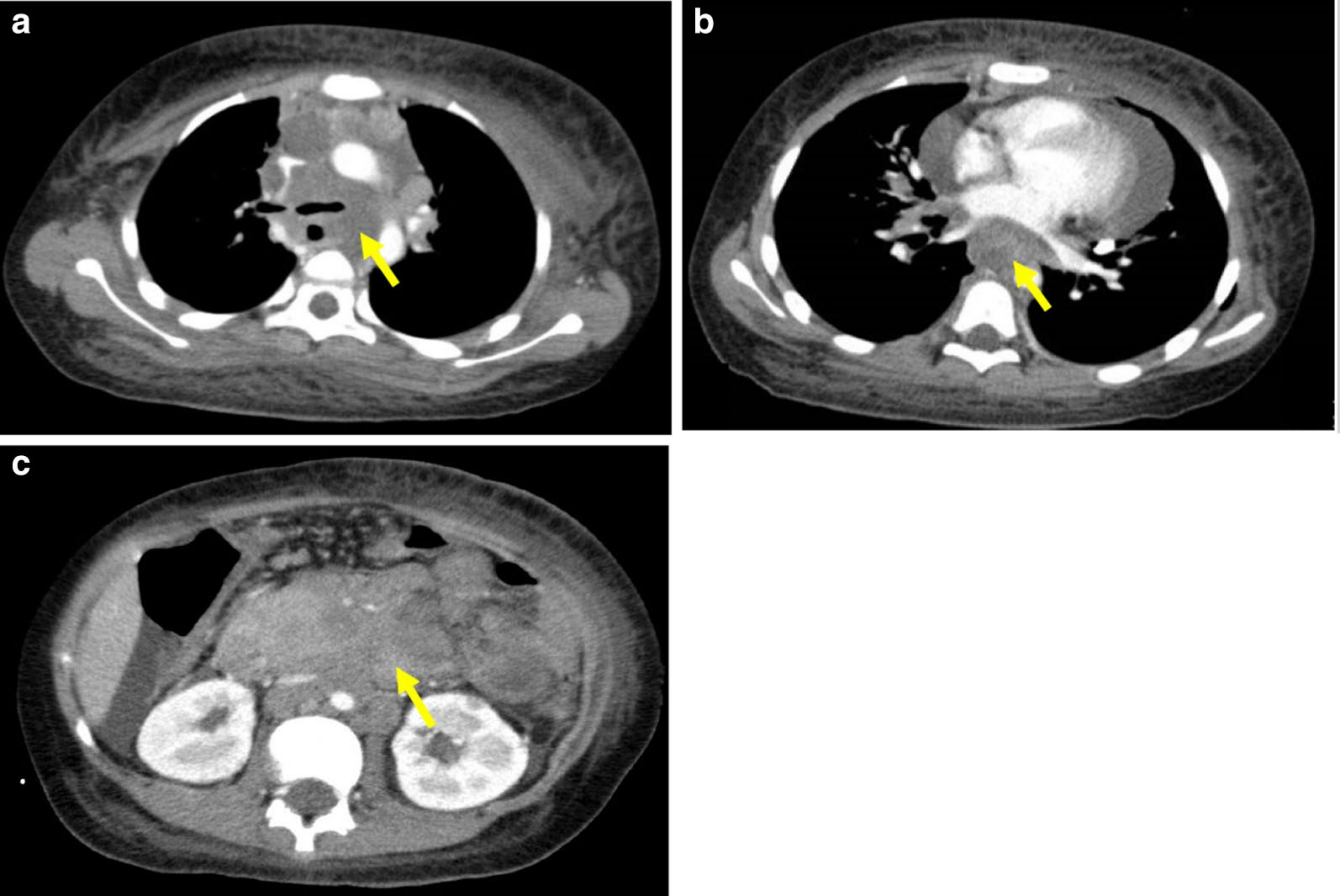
Contrast-enhanced computed tomography (CT) at admission. a Chest CT revealing mediastinal lymphadenopathy along with compression of the left and right bronchi (yellow arrow). b Chest CT revealing mediastinal lymphadenopathy along with compression of the esophagus (yellow arrow). c Abdominal CT revealing lymphadenopathy of the para-aorta, pancreas, and mesentery (yellow arrow)
